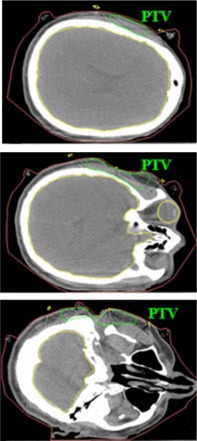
(Color) Transverse CT slices of Patient 1 showing the location, depth, and shape of the parotid PTV at various levels.
